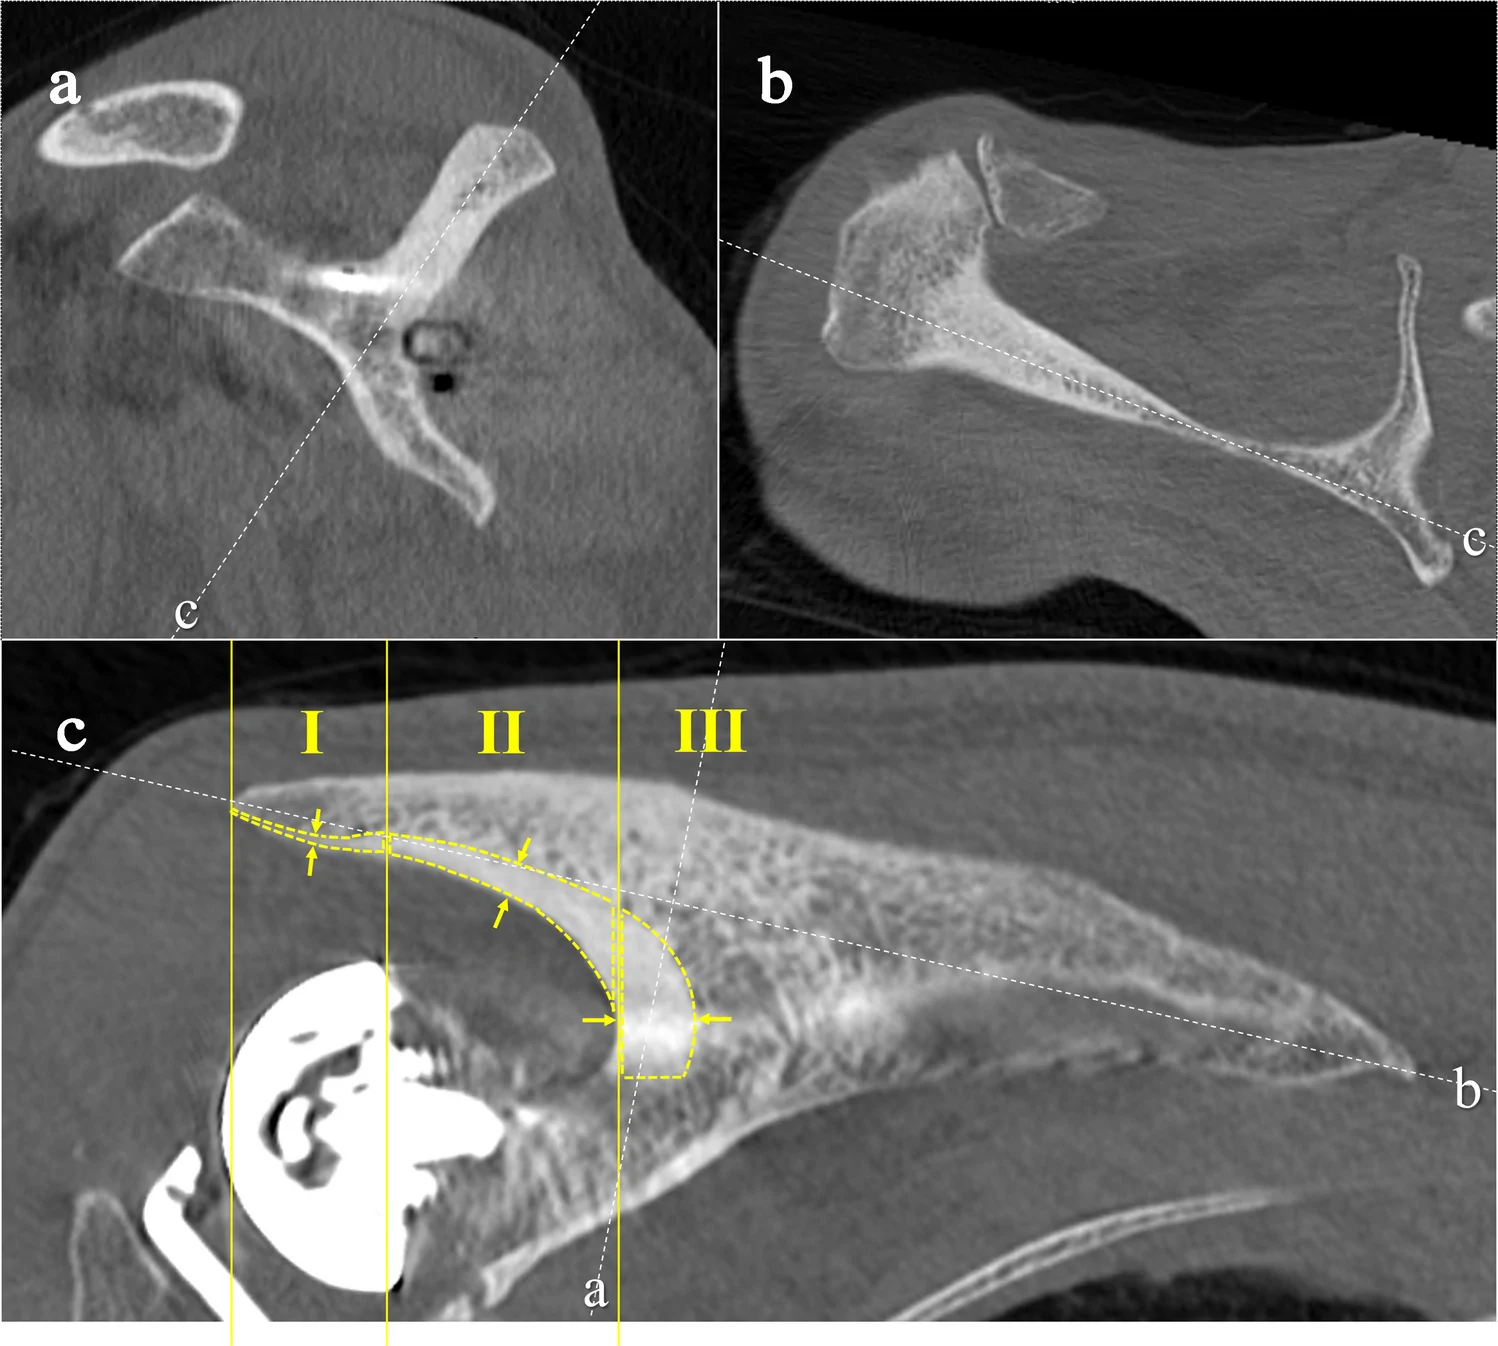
CT reconstruction, zoning, and measurement methods on the acromial coronal view. a, b Sagittal and axial reference planes. Dashed lines indicate the planes used to create the acromial coronal view by multiplanar reconstruction. c Reconstructed acromial coronal view. The acromion was divided into three zones: Zone I, lateral to the centre of rotation; Zone II, between the centre of rotation and the acromial base; and Zone III, medial to the acromial base. The centre of rotation was defined as the centre of the glenosphere. Inferior acromial cortical thickness was measured perpendicular to the cortical surface at the centre of each zone (arrows). Inferior cortical area was measured by tracing the inferior cortical region in each zone as a region of interest (yellow outline)
